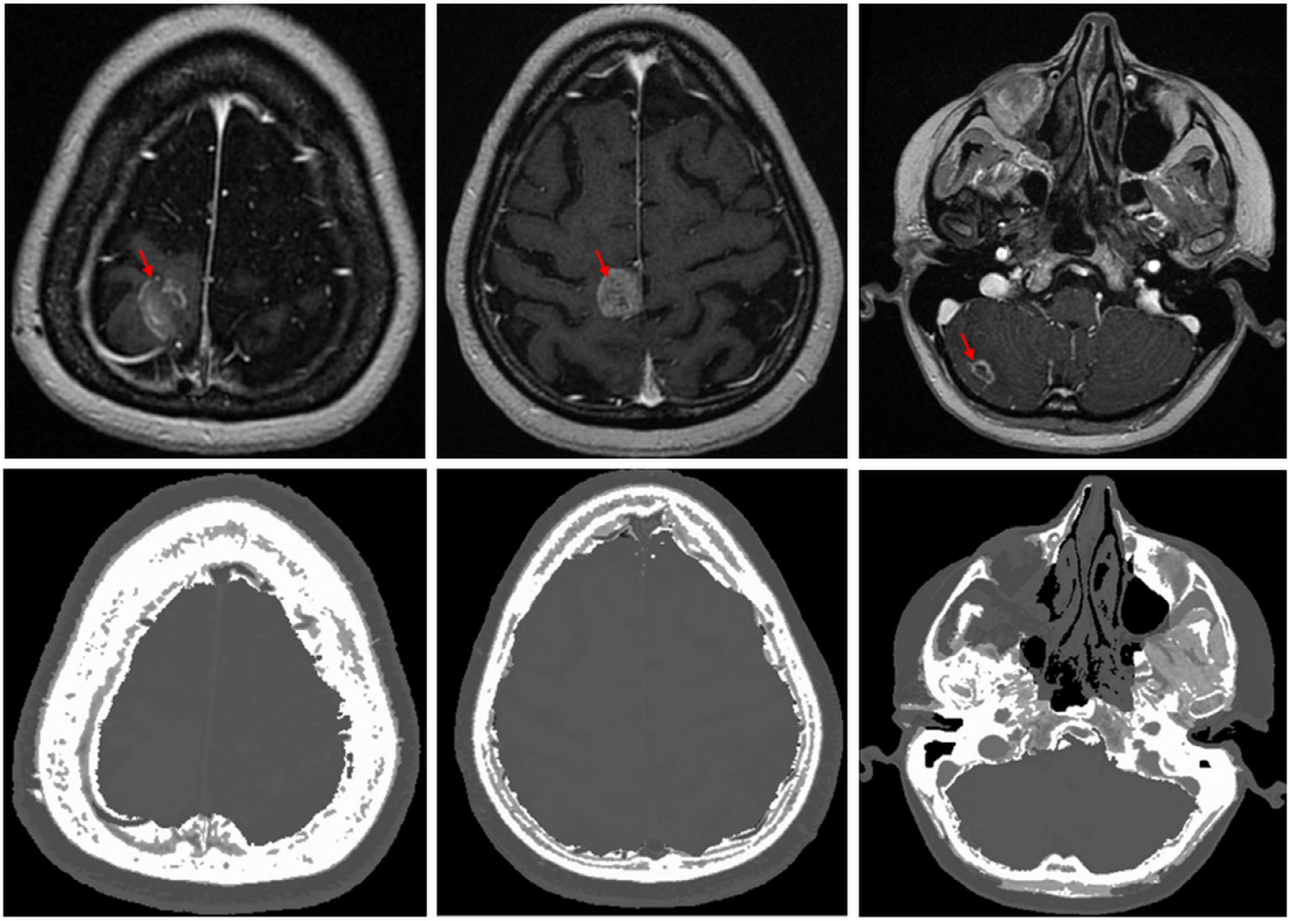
Magnetic resonance slices (upper row) and corresponding synthetic‐CT slices (lower row) for three patients. Left most images show a patient who has undergone surgery and leaky cerebrospinal fluid (left case) and enhanced tumors (red arrows)
